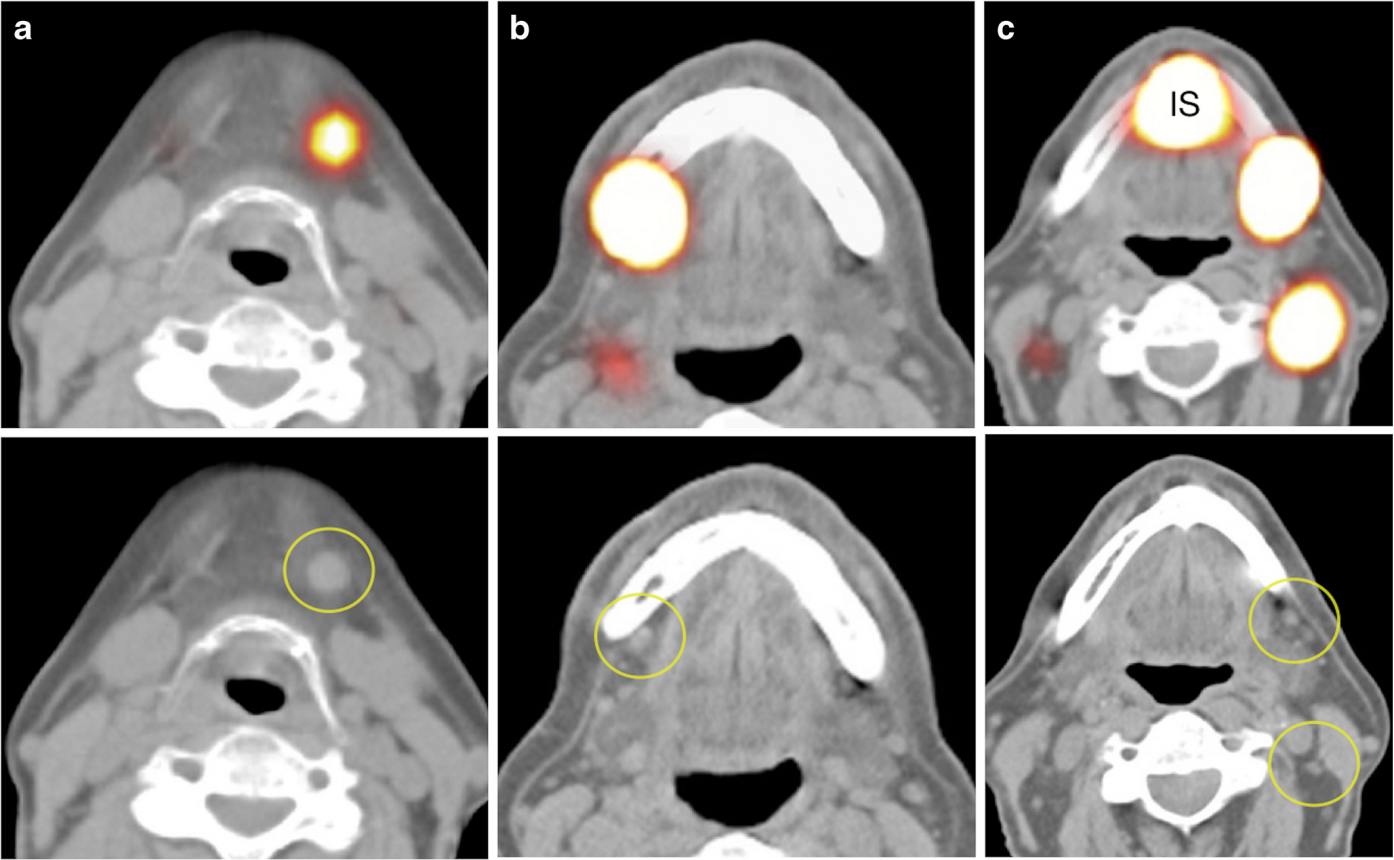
Importance of SPECT/CT and low-dose (ld) CT in characterising sentinel lymph nodes (SLN) in the vicinity of primary tumours in oral cavity. On the *left* (A) SPECT/CT (*top*) showing uptake in a SLN in level 1a on the left, which corresponds with an enlarged lymph node (*circle*) on ldCT (*bottom*). On *middle* (B) SPECT/CT (*top*) shows intense uptake in a SLN in level 1b on the right, whereas on corresponding ldCT (*bottom*) a slightly elongated lymph node (*circle*) is seen. Finally, on the *right* (C) SPECT/CT (*top*) shows drainage from the injection site (IS) to SLNs respectively corresponding with a lymph node cluster in level Ib and a single node in level II of the left side of the neck (*circles*) on ldCT (*bottom*)
